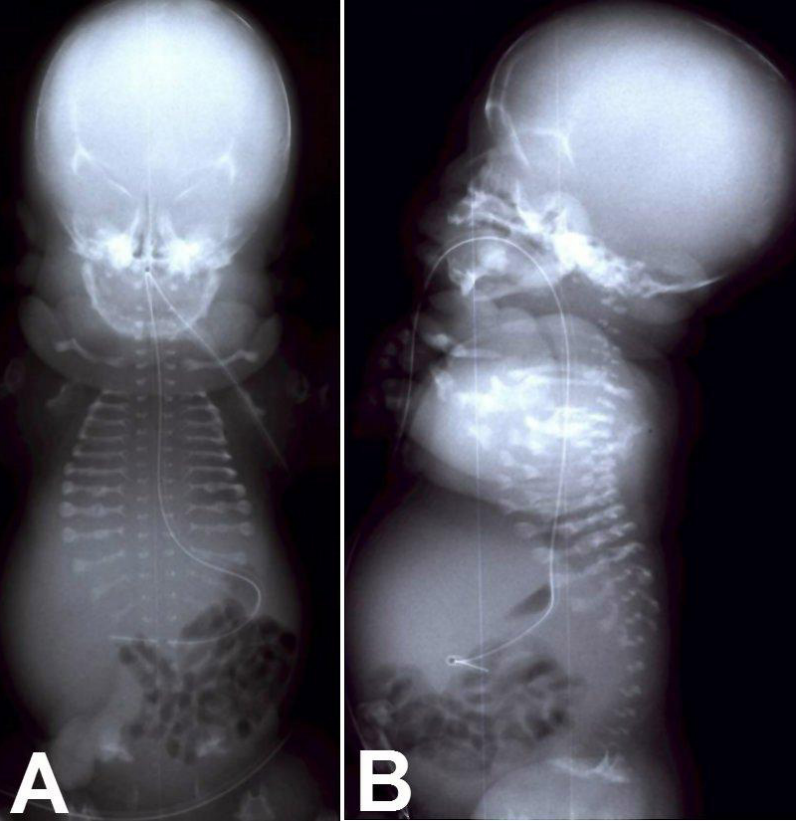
Postmortem anteroposterior (A) and lateral (B) whole-body radiographs of the baby.
